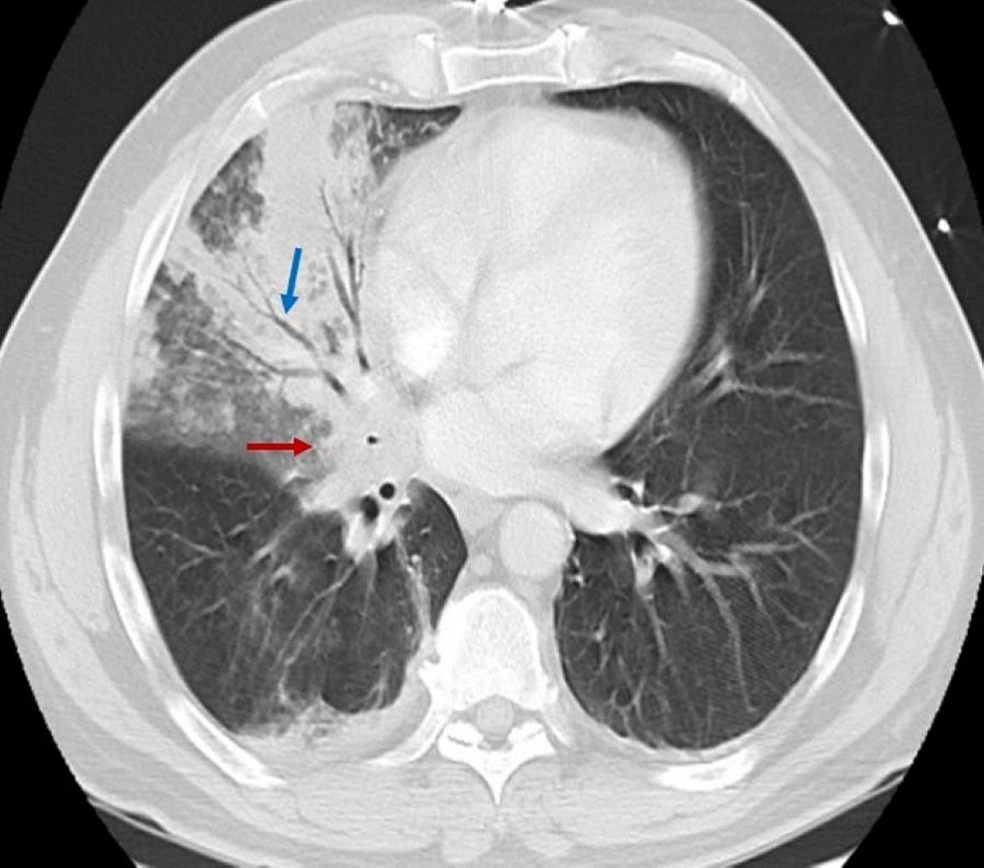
Chest CT depicting patchy airspace disease with air bronchograms (blue arrow) in the right middle lobe and a right infrahilar mass (red arrow) measuring 3.3 × 2.6 cm with encasement of the right middle lobe bronchi. CT: computed tomography
